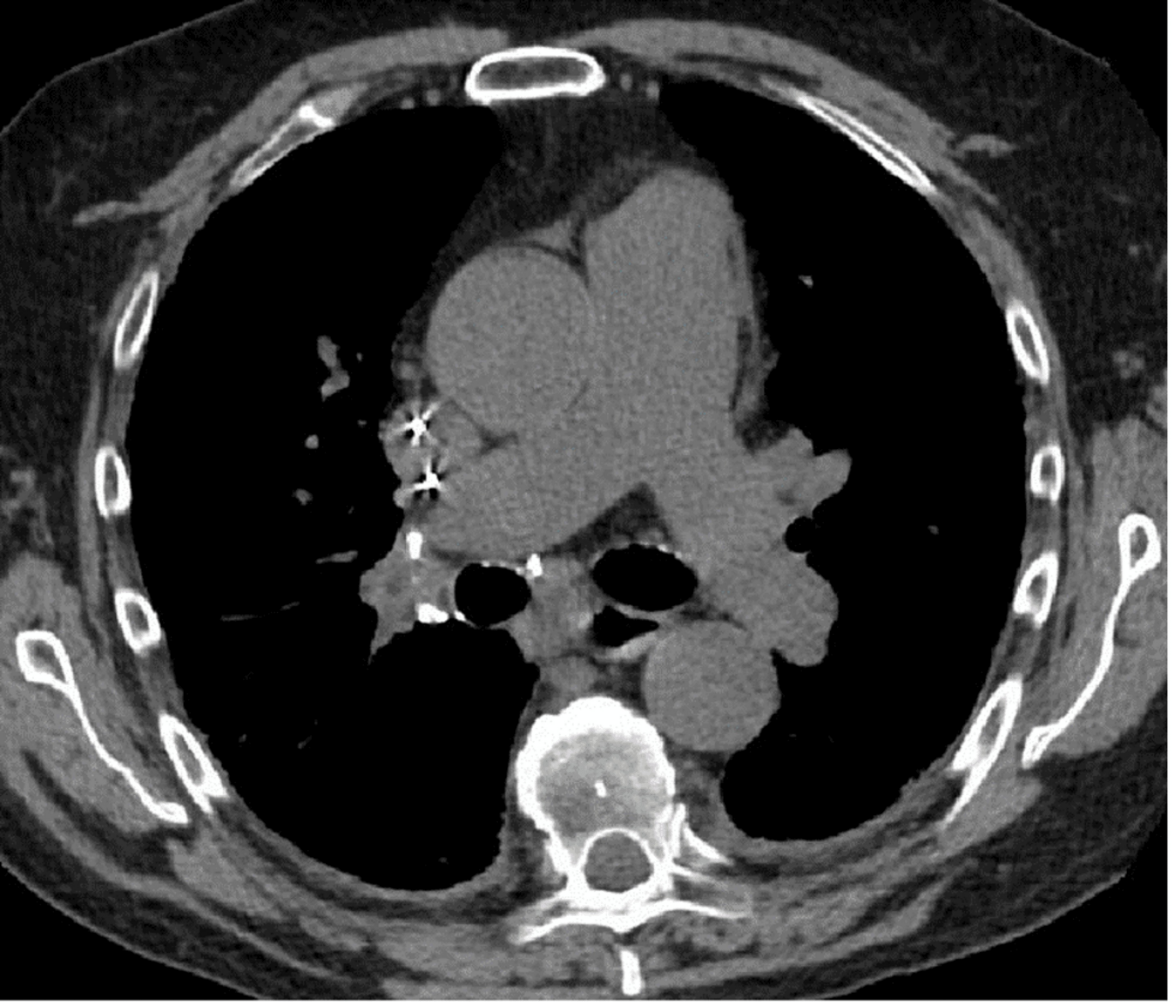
CT chest demonstrating calcified mediastinal lymphadenopathy. CT chest without contrast (soft tissue window) shows bulky, symmetrical calcified mediastinal lymphadenopathy, consistent with long-standing sarcoidosis radiological stage I.
